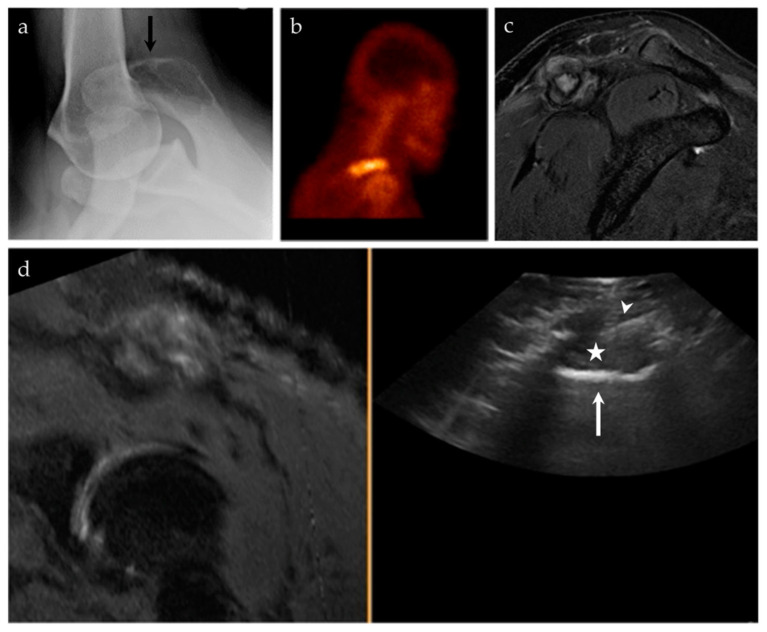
A 45-year-old male with pain at the right shoulder. (a) Axillary view of the right shoulder shows a geographic lytic lesion at the right acromion (black arrow). (b) Nuclear medicine bone scan (^99m^Tc-MDP) shows an area of increased radiotracer uptake at the right shoulder, corresponding to the lytic lesion seen on radiographs. (c) Sagittal T2 fat-saturated sequence of the right shoulder shows a corresponding T2 hyperintense lesion at the right acromion with an associated soft tissue component and surrounding peritumoral edema. (d) Ultrasound-MRI guided fusion was performed for guidance during biopsy. Although the selected MRI reformatted image (left) is degraded, during real-time there was appropriate correlation. The ultrasound image (right) shows the acromion (white arrow), the soft tissue component to this lesion (asterisk), and the biopsy needle (white arrowhead). Pathology results revealed an epithelioid hemangioma.
